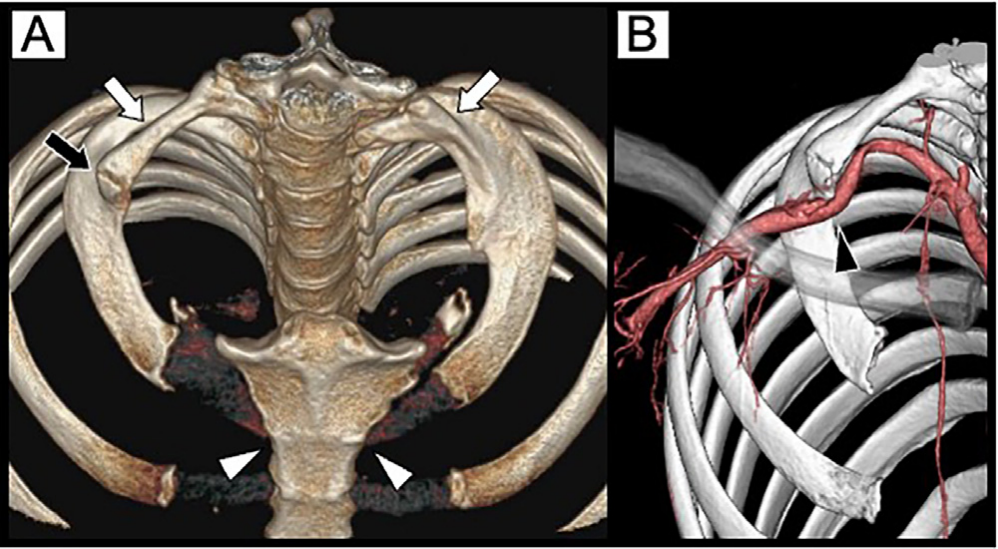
Volume-rendered three-dimensional reconstruction from the computed tomography image. (A) Abnormal bone structures resembling cervical ribs are observed on both sides (white arrows). These do not attach directly to the sternum and, on the right side, are fused with the caudal rib in a pseudoarthrosis-like structure (black arrow). The caudal ribs attach to the second sternal notch (white arrowheads). (B) The right subclavian artery is strongly compressed by the pseudoarthrosis-like structure (black arrowhead).
